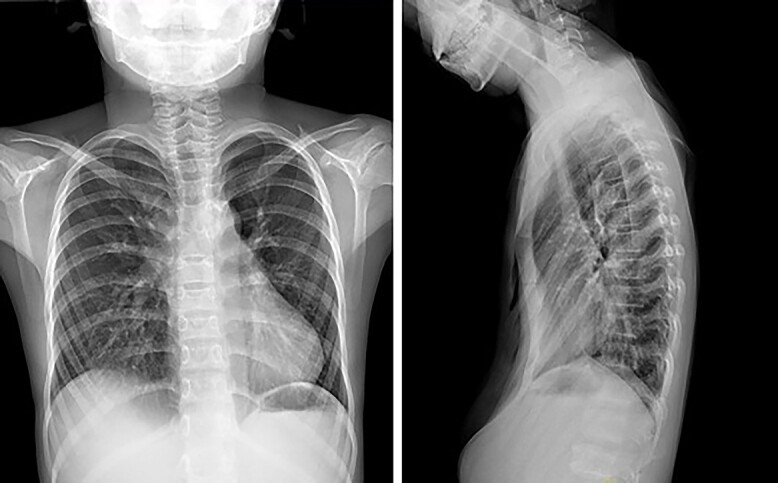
Chest X-ray examination before the first operation.
